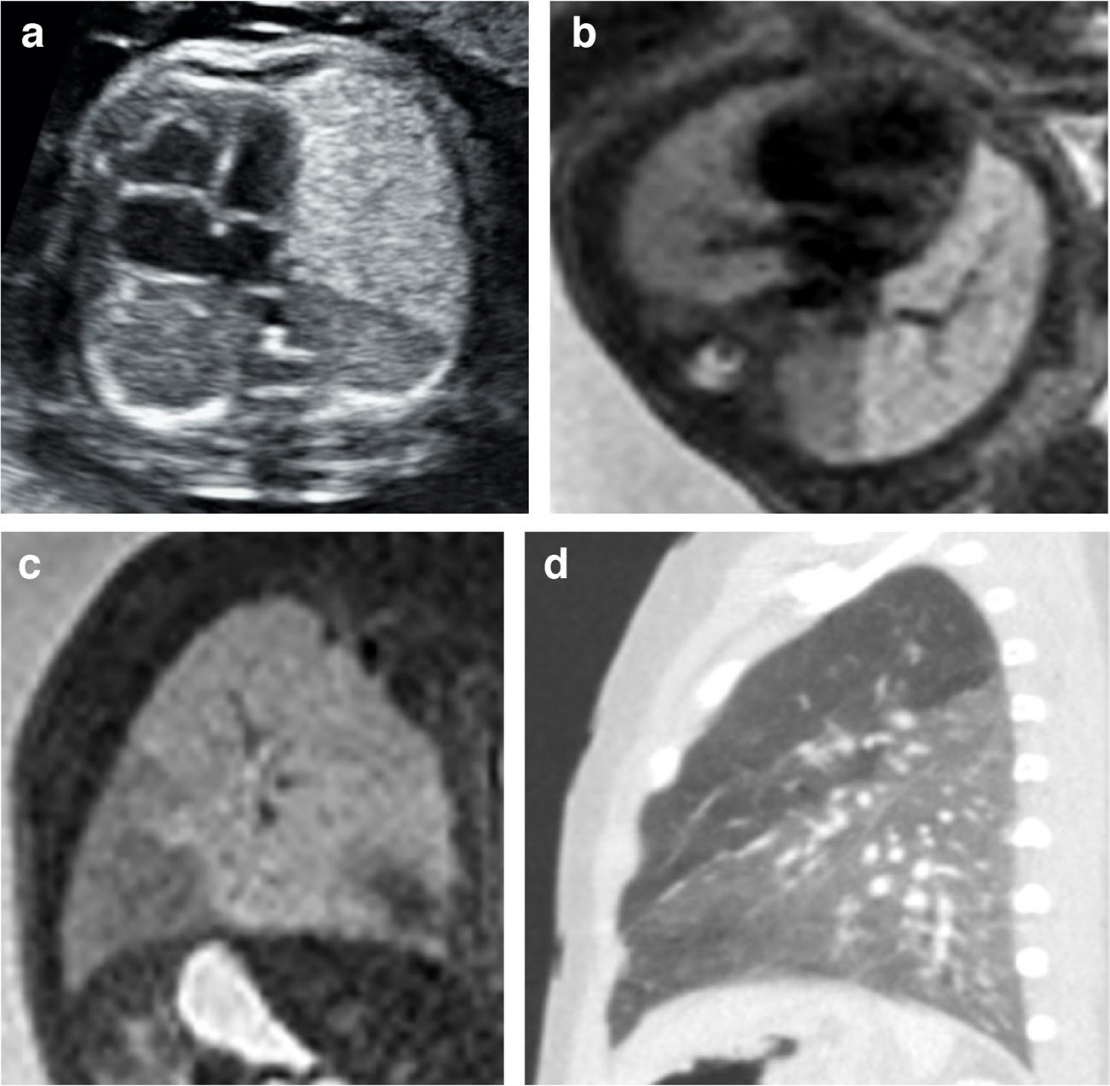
a–c Congenital lobar overinflation in a 29-week gestational age male fetus. Transverse ultrasound image (a) and axial (b) and sagittal (c) T2 single-shot magnetic resonance images (MRI) show asymmetry of the lungs; the left upper lobe is enlarged with hyperechogenicity on ultrasound and homogeneous high T2 signal on MRI. d A sagittal computed tomography image on lung window settings performed at 6 months of age demonstrates a hyperexpanded left upper lobe. Surgery two months later confirmed congenital lobar overinflation
